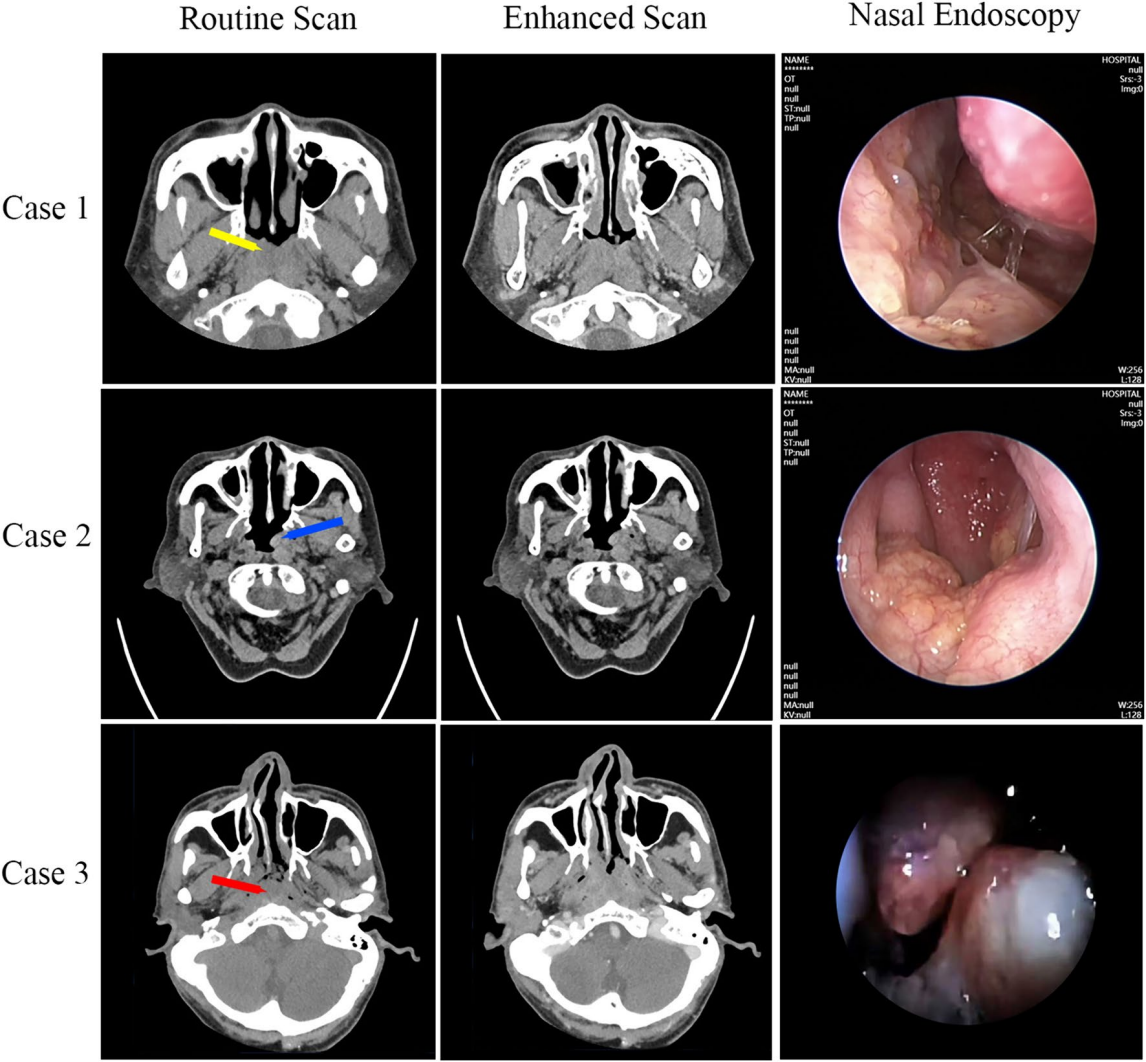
Routine scan, enhanced CT and nasal endoscopy images of three patients. The CT scan revealed low-density, flaky shadows in the nasopharyngeal cavity, with uneven density and an unclear boundary. The enhanced scan displayed mild enhancement and partial non-enhancement. The nasal endoscopy revealed an irregular, gray-red mass obstructing the nasopharyngeal cavity, accompanied by a rough mucosal surface
